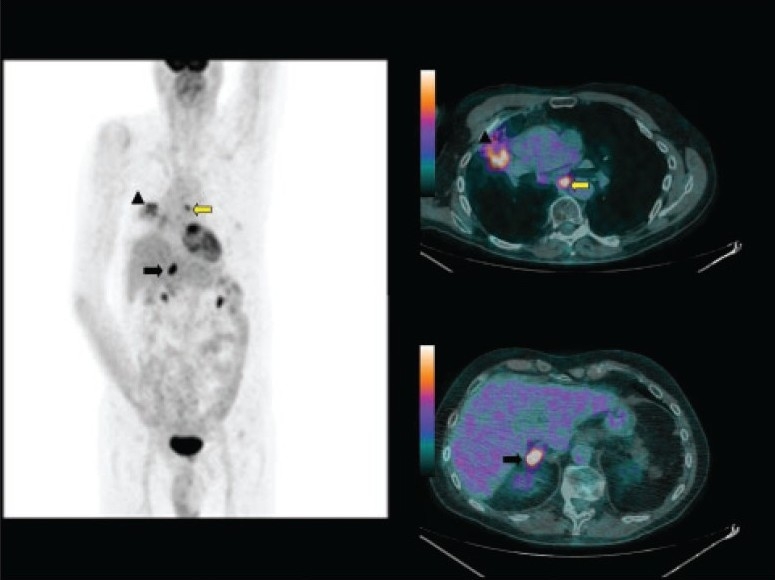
A 68-year-old man who came for initial staging of non-small cell lung cancer. MIP image (left panel) and fused images (right panel) of 18F-FDG PET/CT showed the primary tumor (arrow head) with mediastinal nodal involvement (yellow arrow) and extra-thoracic right adrenal metastasis (black arrow).
